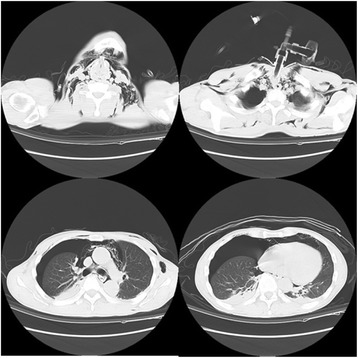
CT images of the neck and thorax on the day following tracheostomy. CT images revealed emphysema of the chest, neck and mediastinum, and pneumothorax of the right lung
